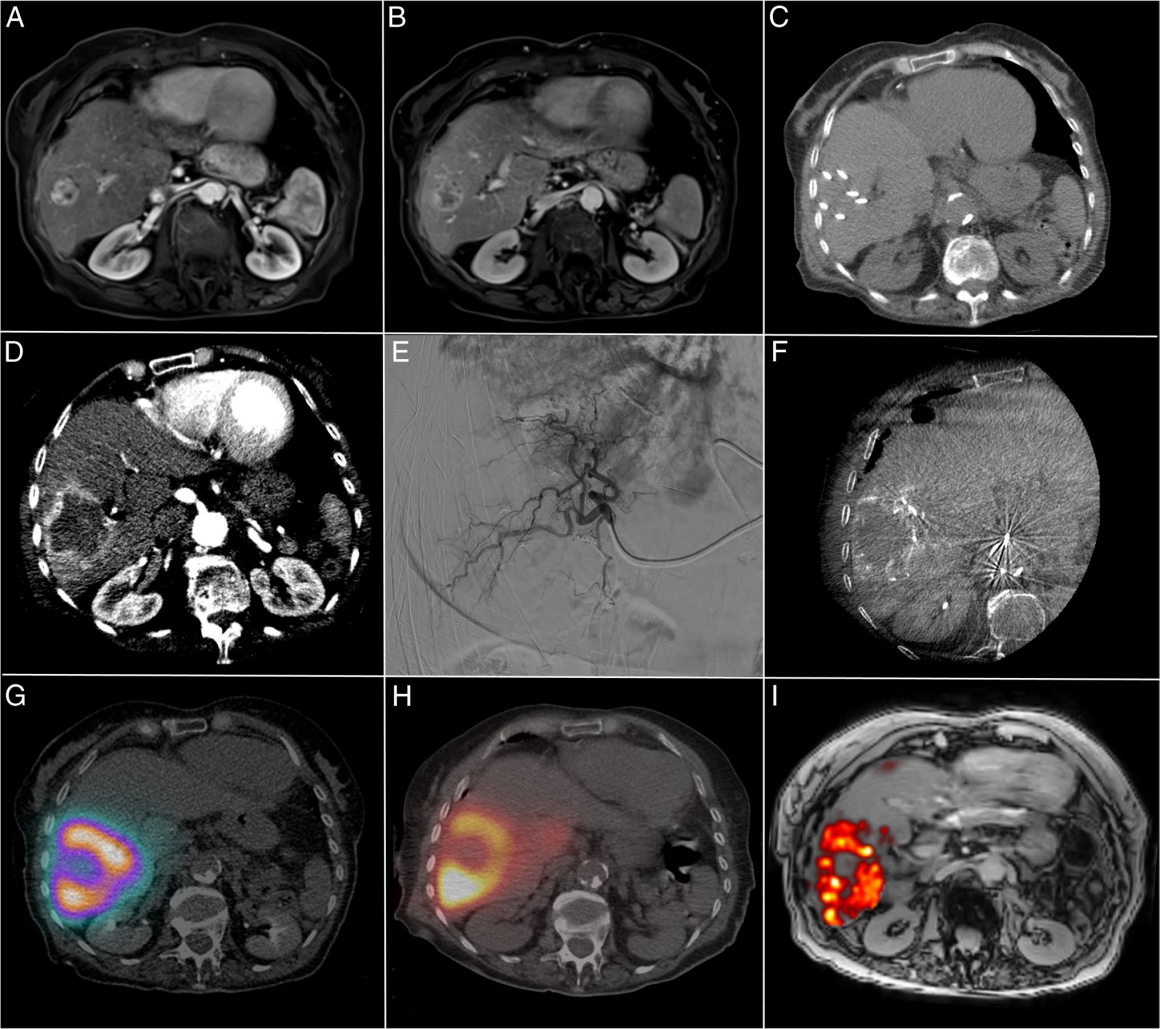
HORA EST HCC treatment sequence: A Arterial scan phase of diagnostic MRI showing a hypervascular HCC lesion of 31 mm in the liver. B Portal venous scan phase of MRI showing central wash-out in the HCC lesion. C Intraprocedural CT after placement of six cooled-tip RFA needles with 3-cm exposed tip. D Intraprocedural contrast enhanced CT scan in arterial phase showing hyperemia around the ablation zone on post-ablation CECT. E Super-selective catheterization of hepatic arteries with vascular supply to the target volume. F CBCT of the treatment volume with an identical catheter position as in E. G SPECT-CT of [^99m^Tc]Tc-MAA dose distribution used for dose planning. H SPECT-CT of ^166^Ho-MS distribution. I MRI-based dosimetry of ^166^Ho-MS distribution.
